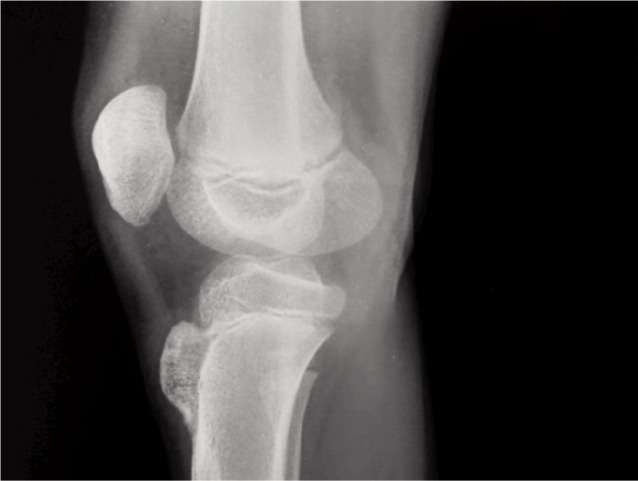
Radiograph of the right knee lateral view shows a bony mass arising from the tibial tuberosity.
